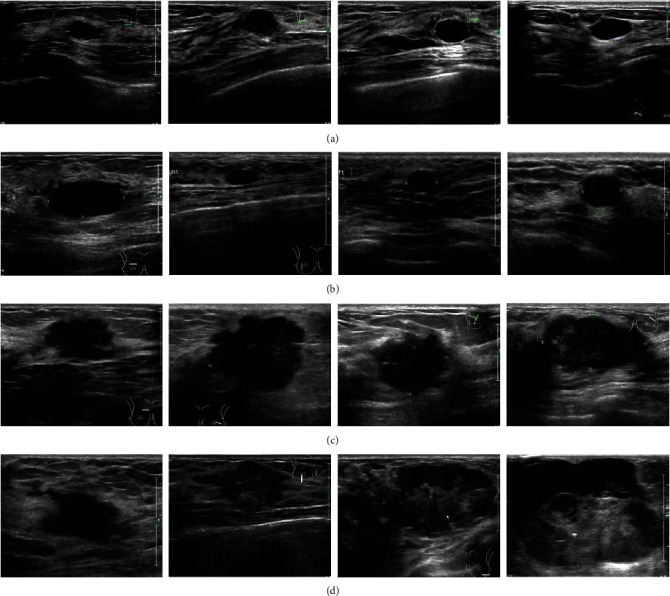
Samples of ultrasound images of breast tumors classified according to BI-RADs standard: (a, b) are benign tumors and (c, d) are malignant tumors. Benign tumors are usually well-defined and round or oval in shape. Malignant tumors are usually poorly defined and irregular with lobules.
